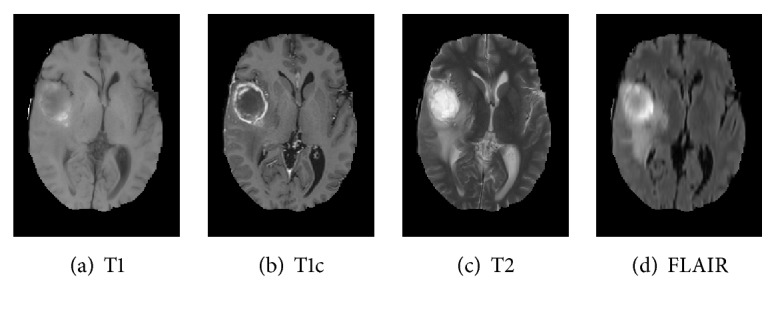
Brain MRI images containing tumors in four different modalities.
